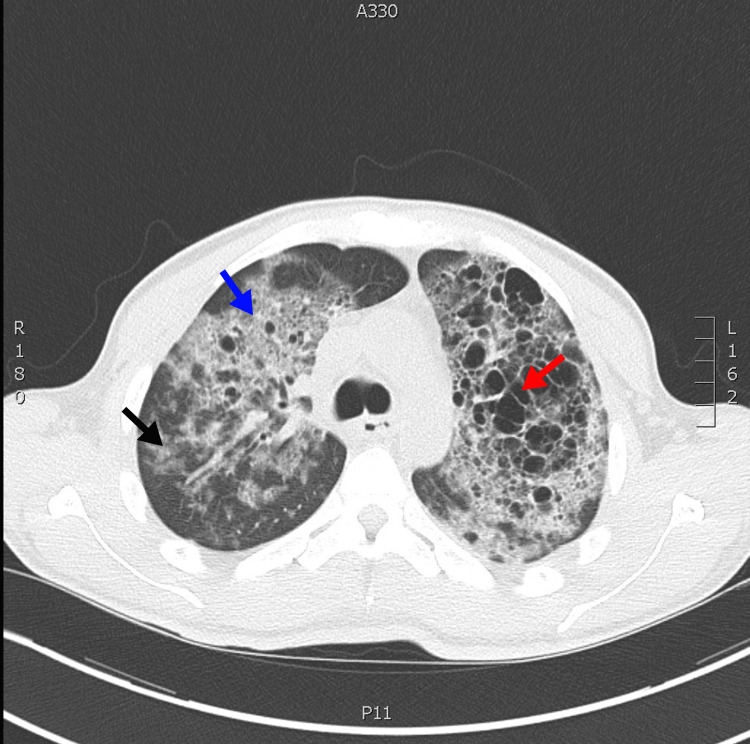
Computed tomography of the chest showed extensive ground glass opacities (black arrow) with consolidative and nodular features (blue arrow) present in both lungs with areas of cystic proliferation (red arrow). Upper lobe predominance noted
